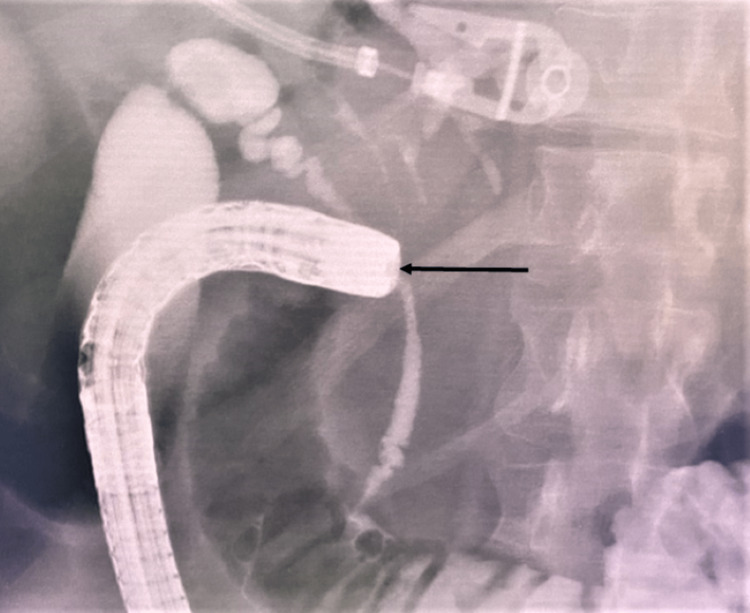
Radiograph showing the passage of the contrast into the biliary tract during injecting the contrast through the fistula.
